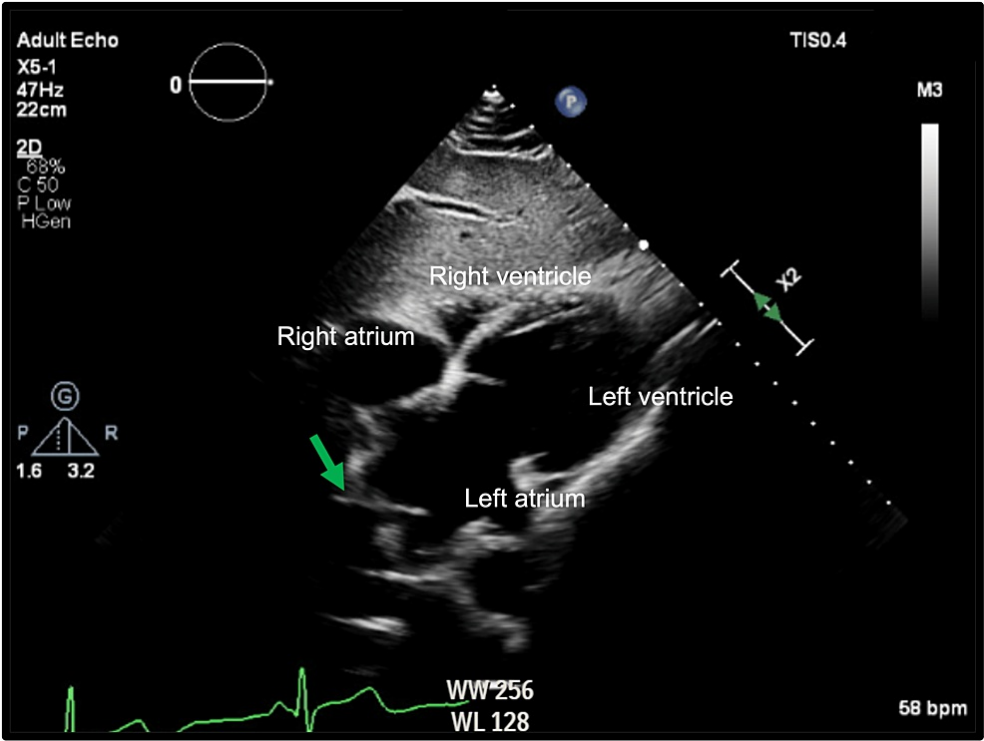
Transthoracic echocardiogram subxiphoid view demonstrating the baffle repair conduit joining the left atrium (green arrow).
